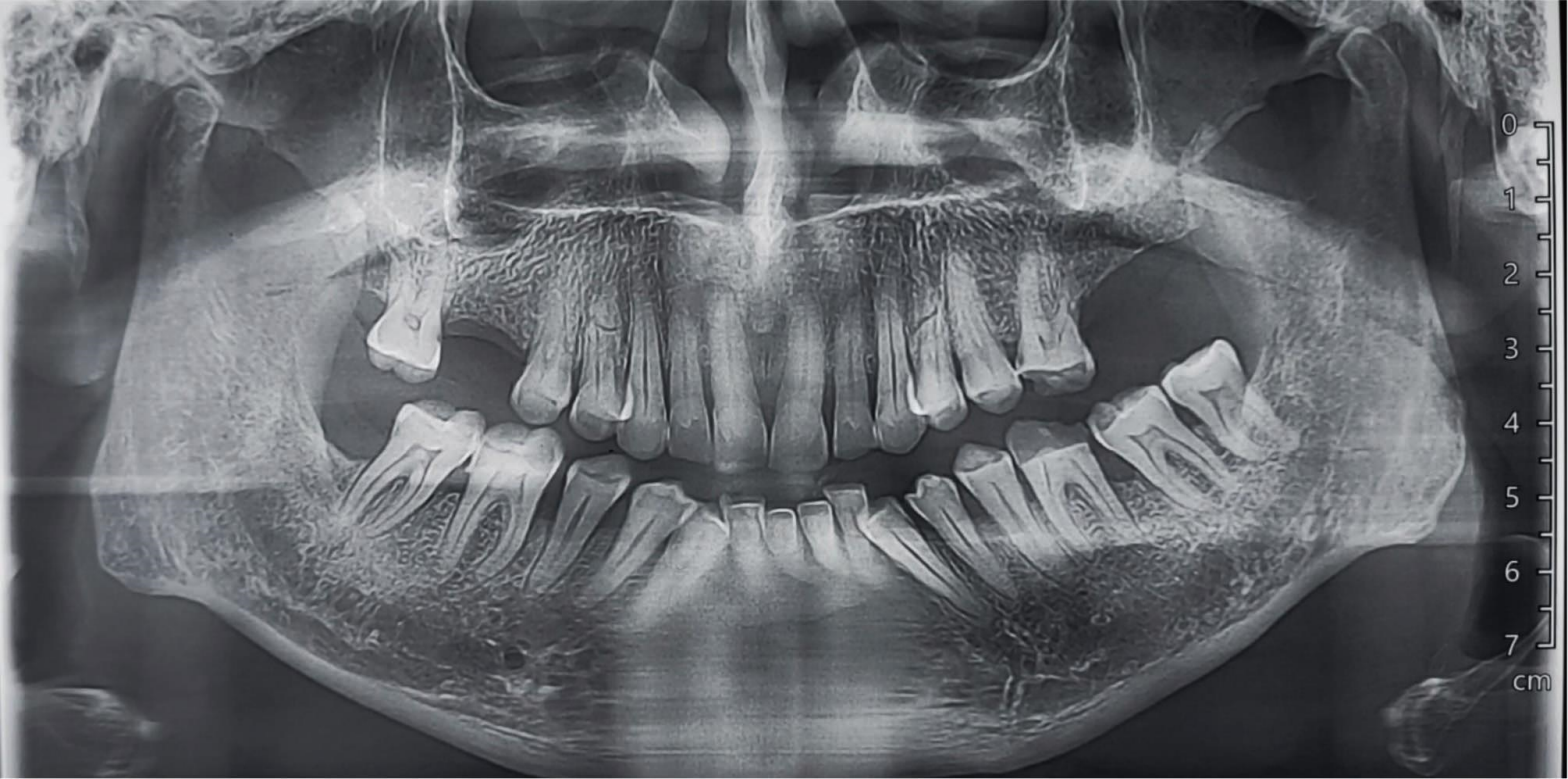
Orthopantomogram (Failed to disclose any abnormalities).
